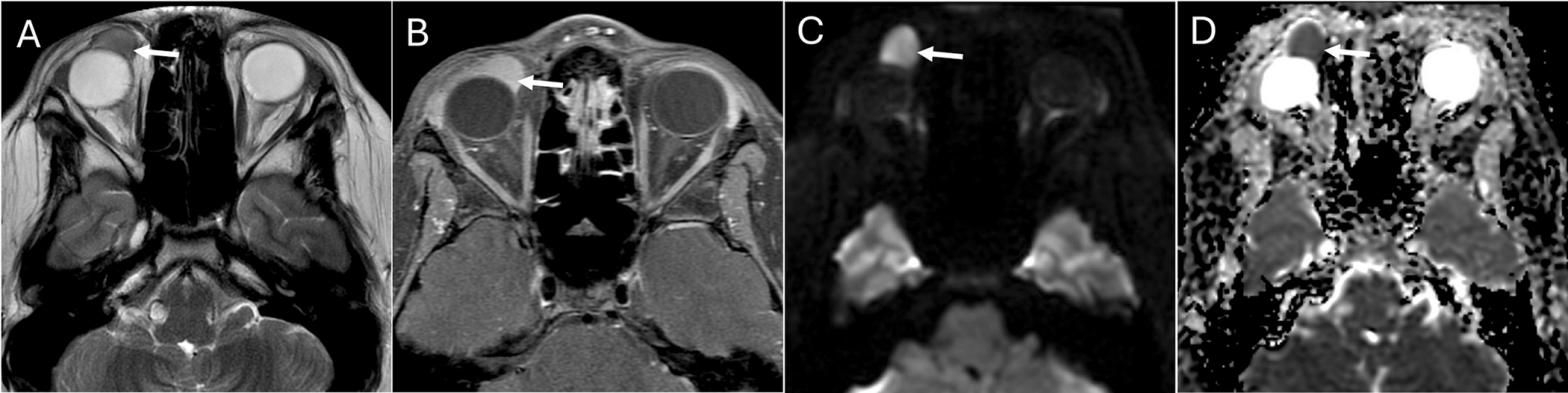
Orbital neoplasm. Axial T2 (A) and post-contrast T1 fat saturated (B) images in a 24 year-old male show a round hypointense and homogeneously enhancing preseptal mass abutting the globe (white arrows). No surrounding inflammatory fat stranding, swelling, or contralateral abnormality is noted. Axial diffusion-weighted imaging (DWI) (C) shows hyperintense signal compared to white matter with corresponding relatively low signal on apparent diffusion coefficient (ADC) map (D) in the mass, suggesting a cellular neoplasm and favoring lymphoma. Both radiologists agreed on neoplasm. The confirmed pathologic diagnosis of this case was mucosa-associated lymphoid tissue lymphoma.
